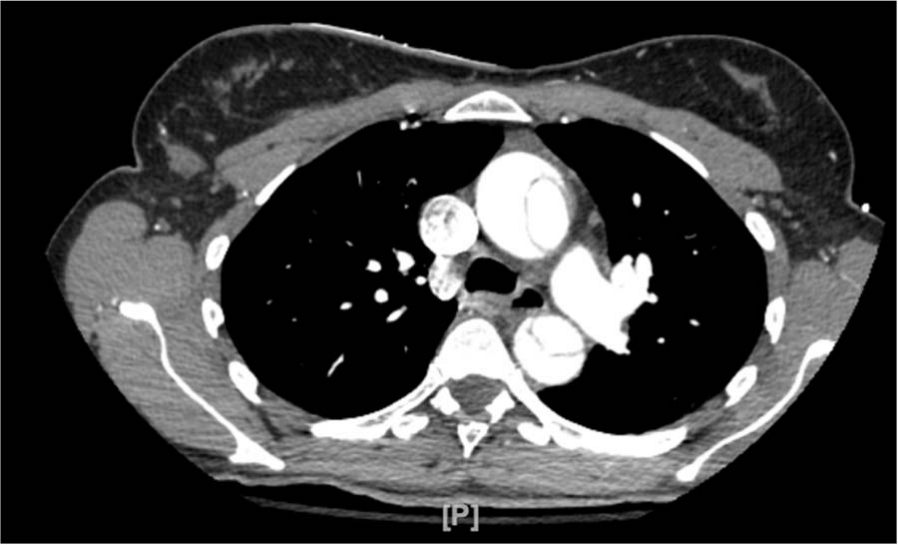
CTA demonstrating type A aortic dissection, with false lumen present in both the ascending and descending portions of the aorta.
